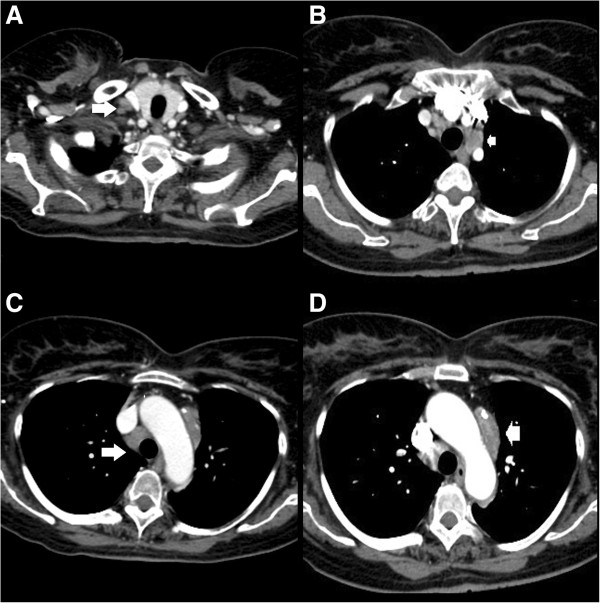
CT scan of mediastinum-axial section. CT scan of mediastinum shows diffuse lymphatic swelling (arrow) over right supraclavicular (A), pre-vascular (B-D) and aortopulmonary (C) station with inhomogeneous enhancing after contrast media, suggestive for lymphoma.
